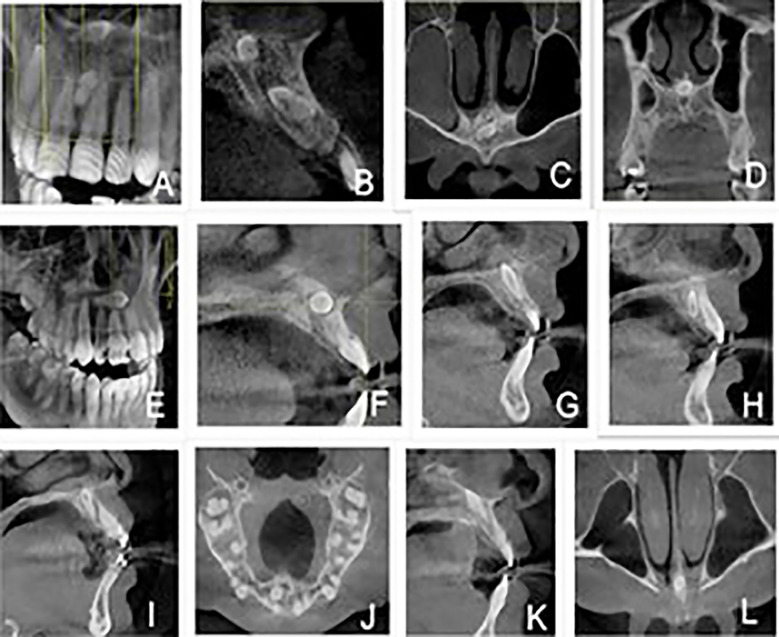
Cone Beam CT Images Depicting Variations in Mesiodens Presentation Including Number, Orientation, Location and Anatomical Relations
